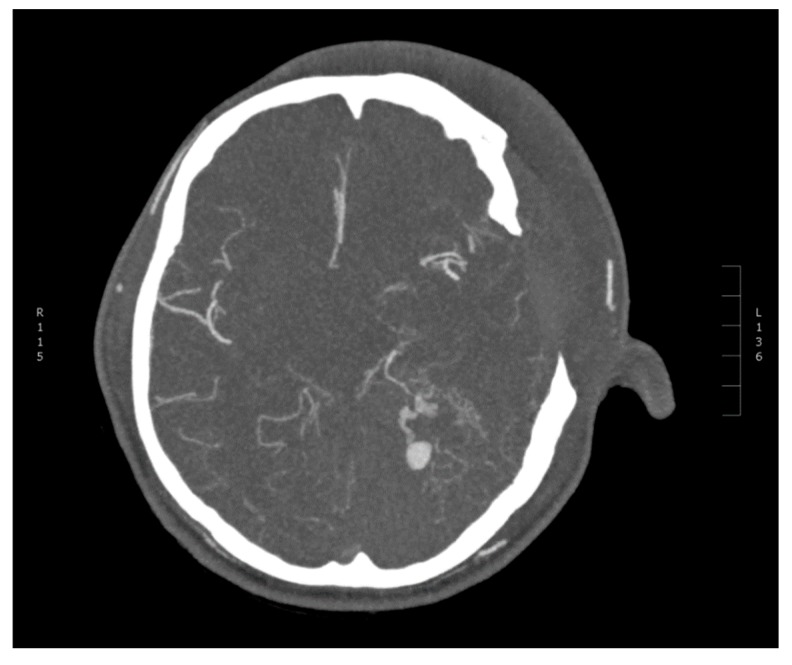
CT angiography of cerebral arteries. Axial maximum intensity projection (MIP) showing a spot sign close to the left tentorium cerebelli.
